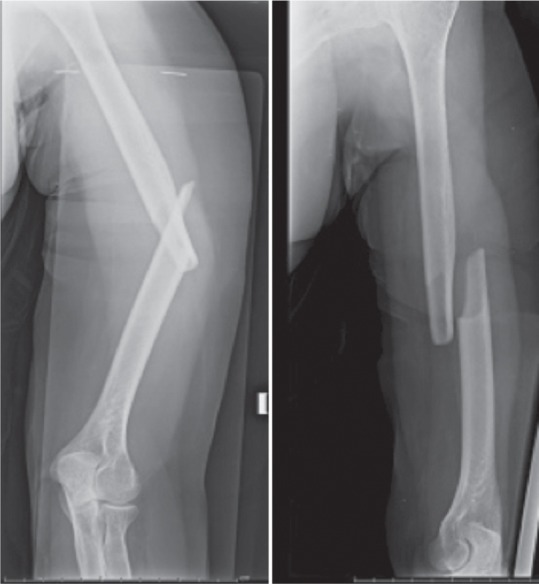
Anteroposterior and lateral radiographs showing fracture middle third shaft of humerus.
